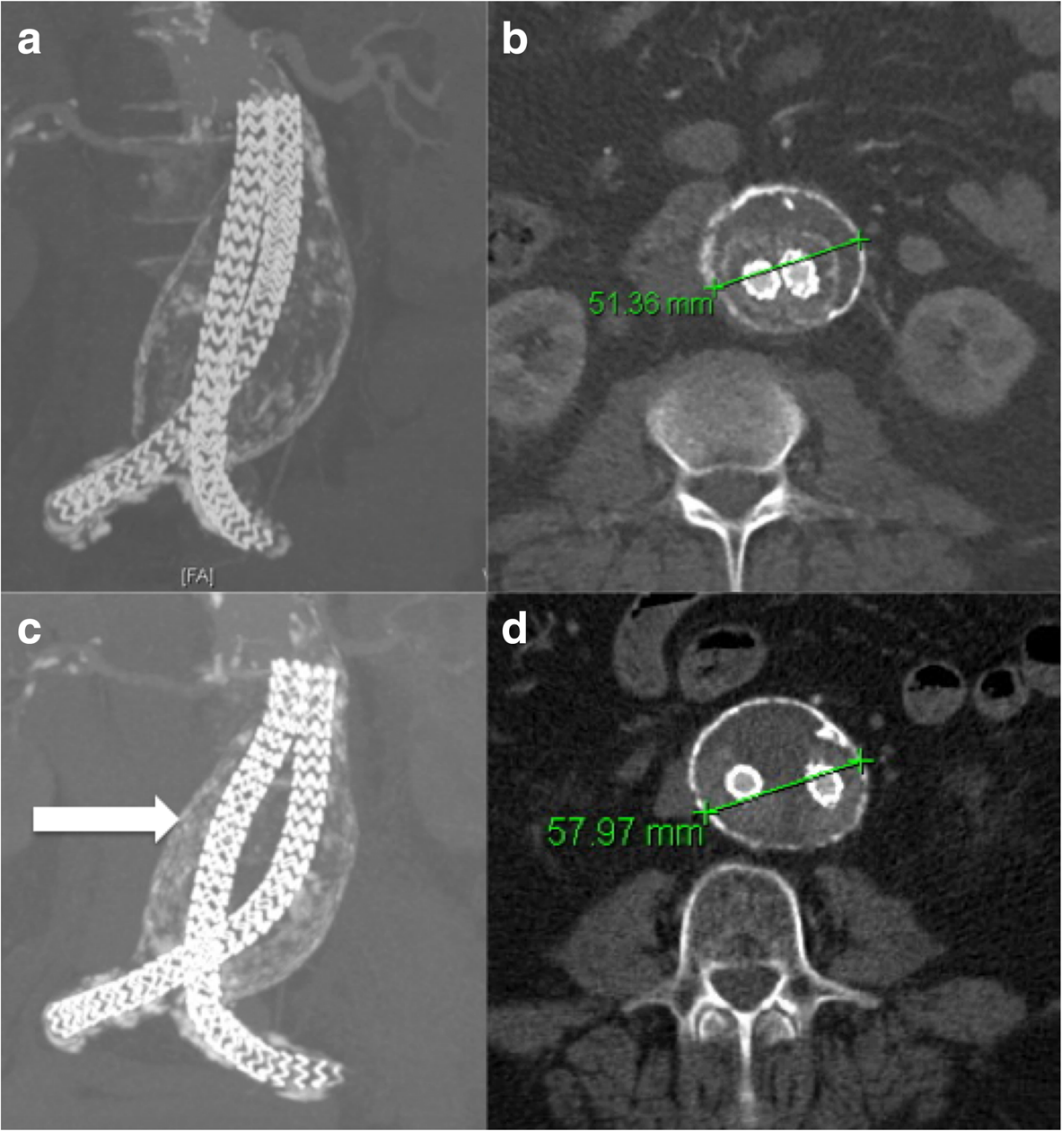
a Coronary reconstruction of a CT scan showing the satisfactory deployment of a Nellix device with lack of separation of the grafts. b Transverse CT scan confirming the sac size after deployment. c, d Follow-up CT 2 years later shows separation of the grafts and sac expansion. This is a result of a subtle type Ia endoleak between the two grafts.
